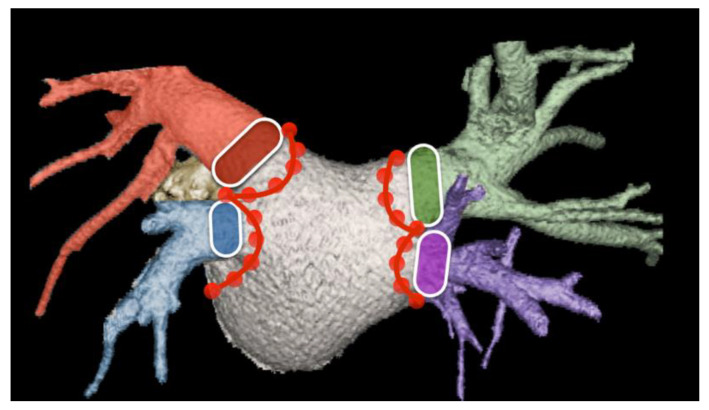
Volume-rendered CT image of the left atrium and pulmonary veins. The four pulmonary veins can be seen draining into the left atrium. The lined oval structures indicate that the myocardial sleeves contain potential arrhythmogenic cells. The principle of this treatment technique is the electrical isolation of these myocardial sleeves from the rest of the left atrium by catheter-induced scar tissue surrounding the ostia of the pulmonary veins (dotted right lines). Therefore, it is important to be aware of any anatomical variation in order to isolate all draining veins.
